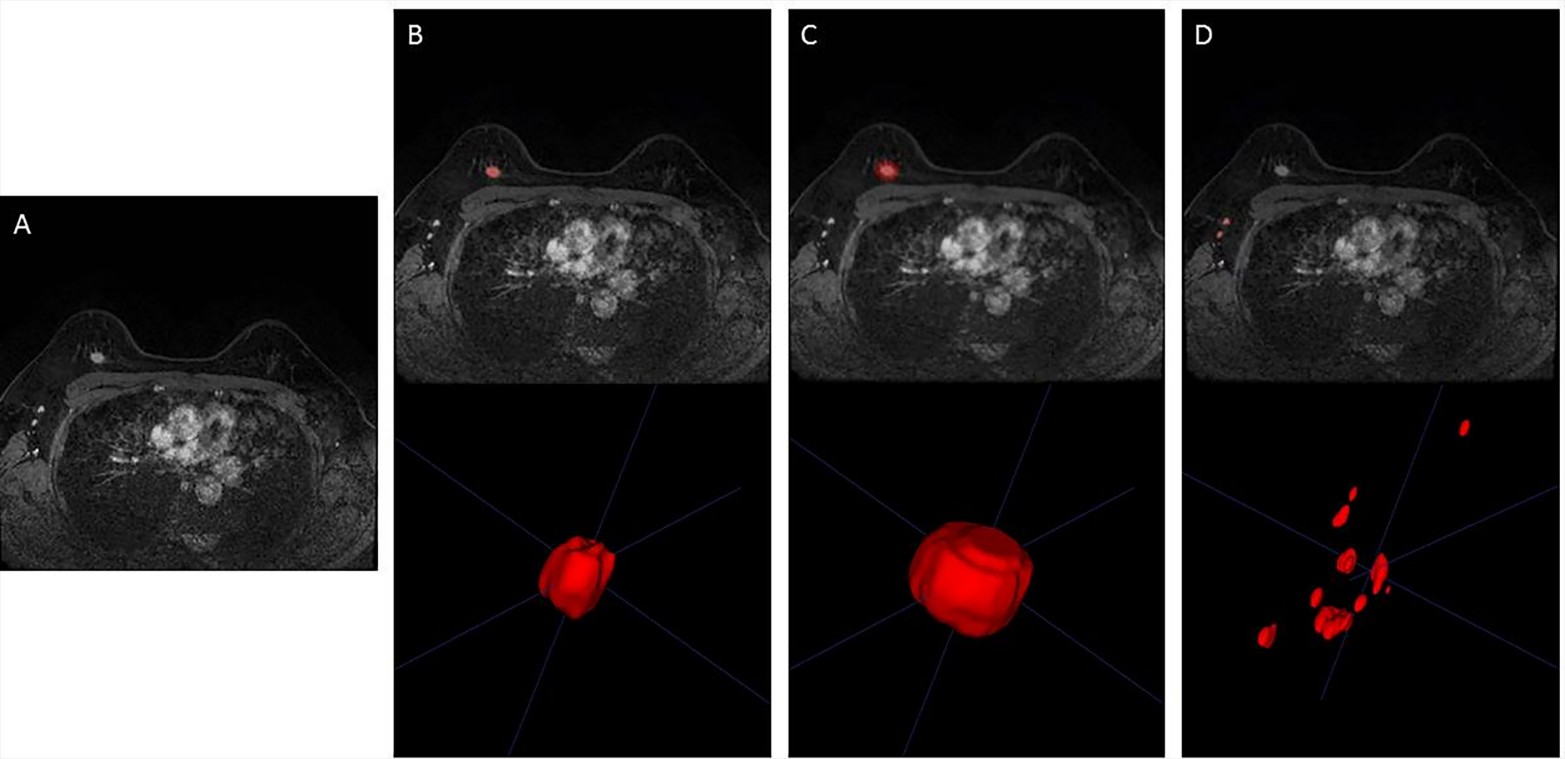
Representative image segmentation. (A) DCE-MRI of a 48-year-old woman with breast cancer in the third phase. (B) Segmentation of breast tumor (ROI of Model 1). (C) Segmentation of intra- and peri-breast tumor (ROI of Model 2). (D) Segmentation of ALN (ROI of Model 3). DCE-MRI, dynamic contrast-enhanced MRI; ROI, region of interest; ALN, axillary lymph node.
